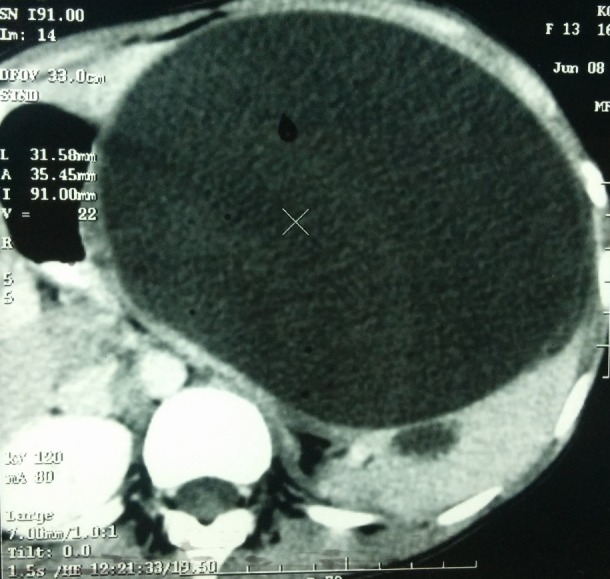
Figure 1:CT scan demonstrating a very large, homogeneous splenic cyst compressing the normal splenic parenchyma and adjacent viscera and even crossing the midline.
